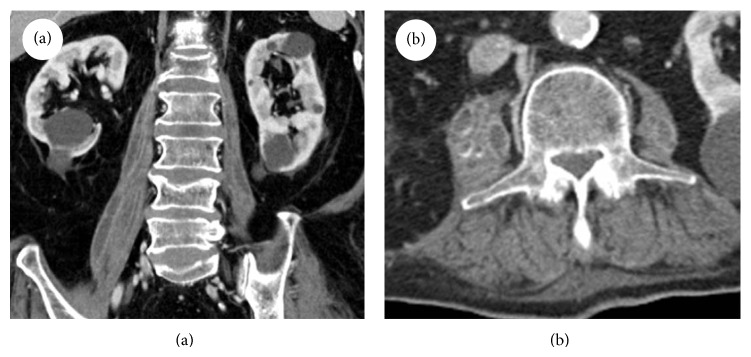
Follow-up abdominal computed tomography showed bilateral multiple abscess formation in the psoas muscle. (a) Frontal plane, (b) Transverse plane.
